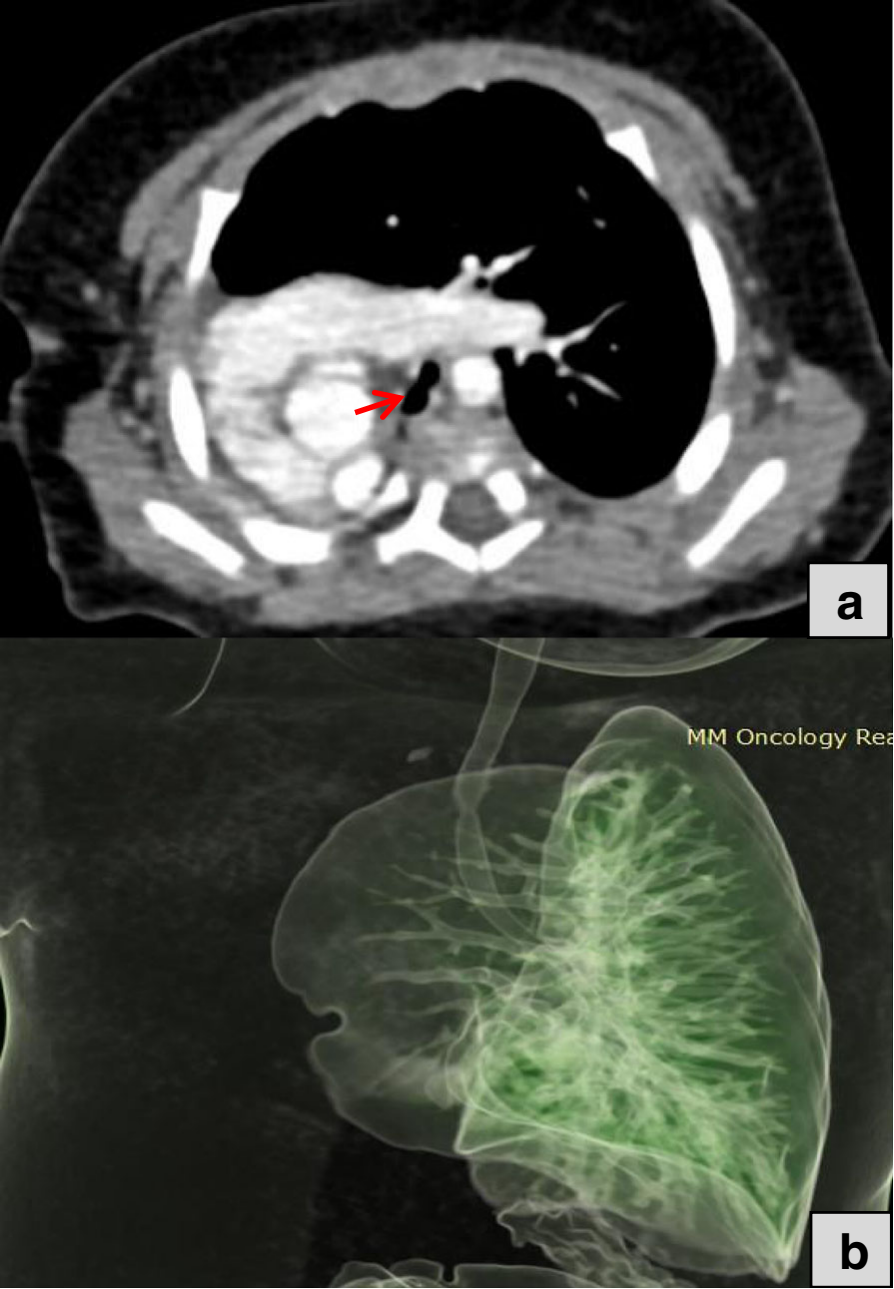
a Axial CT image of three months old baby in mediastinal window shows absent right lung with rudimentary right main bronchus (red arrow) associated with right cardiac and great vessels position with extension of left lung towards the right side. Fig. 3:b Volume rendered image shows unilateral left lung with complete absent right lung and its bronchus in seven months baby
